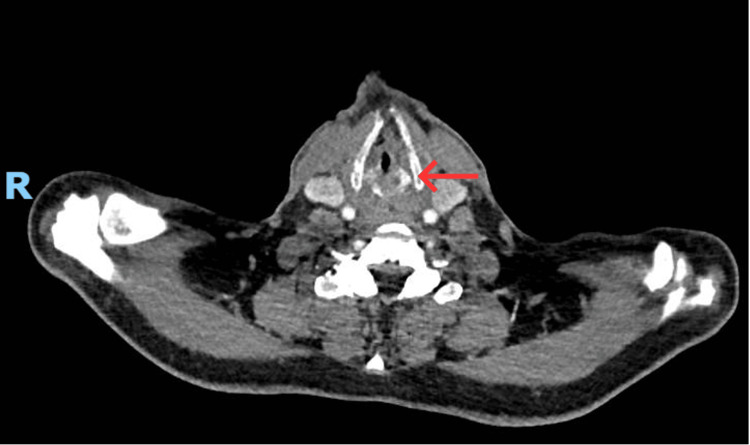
Axial CT of the neck at the level of the thyroid cartilage obtained during stridor, demonstrating bilateral vocal cord, laryngeal and hypopharyngeal oedema. The red arrow highlights circumferential soft-tissue swelling causing marked airway narrowing.
